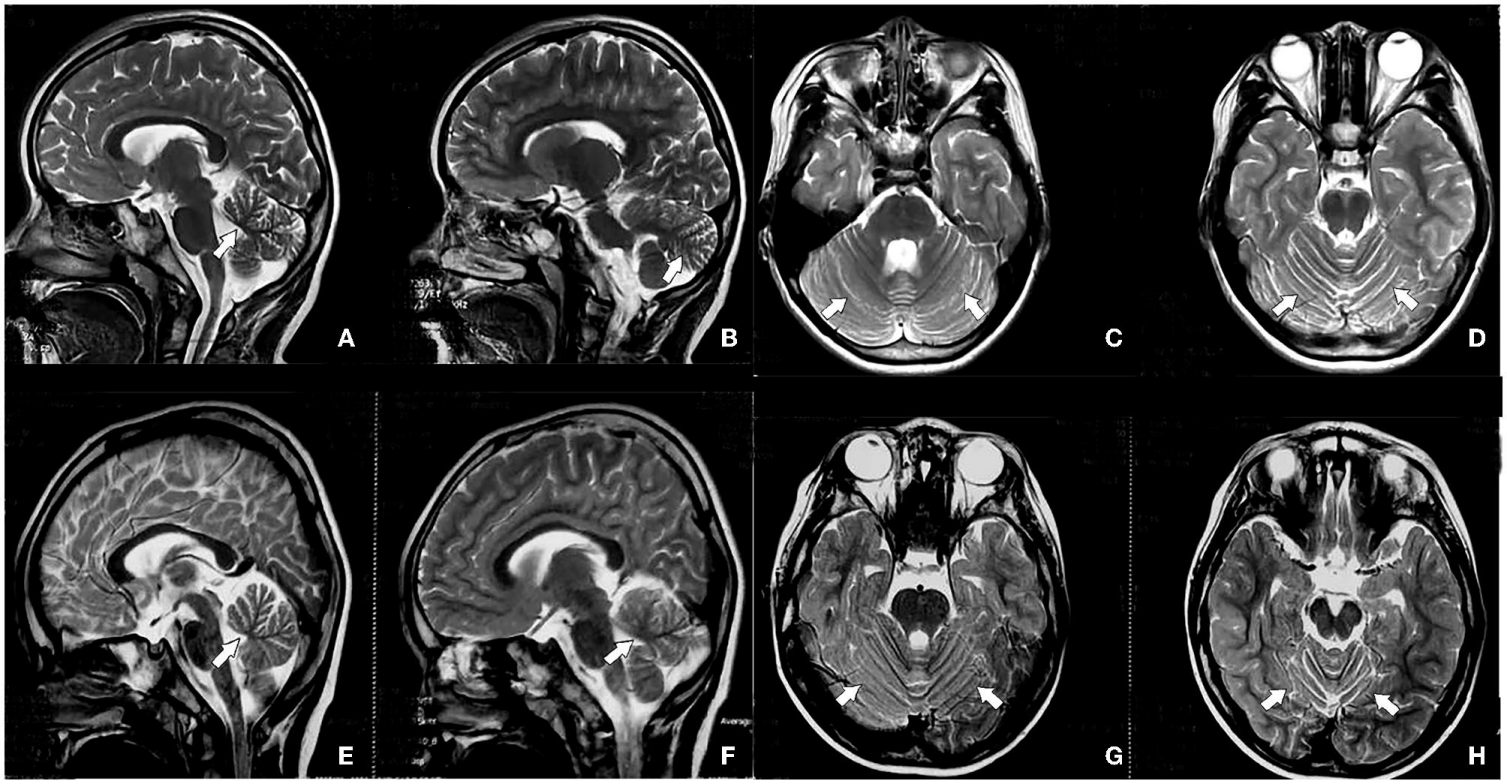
Head magnetic resonance imaging (MRI) of the progenitor (IV.1) shows cerebellar atrophy (A–D), and head MRI of the brother of the predominant (IV.2) shows cerebellar atrophy (E–H), indicated by the white arrow.
